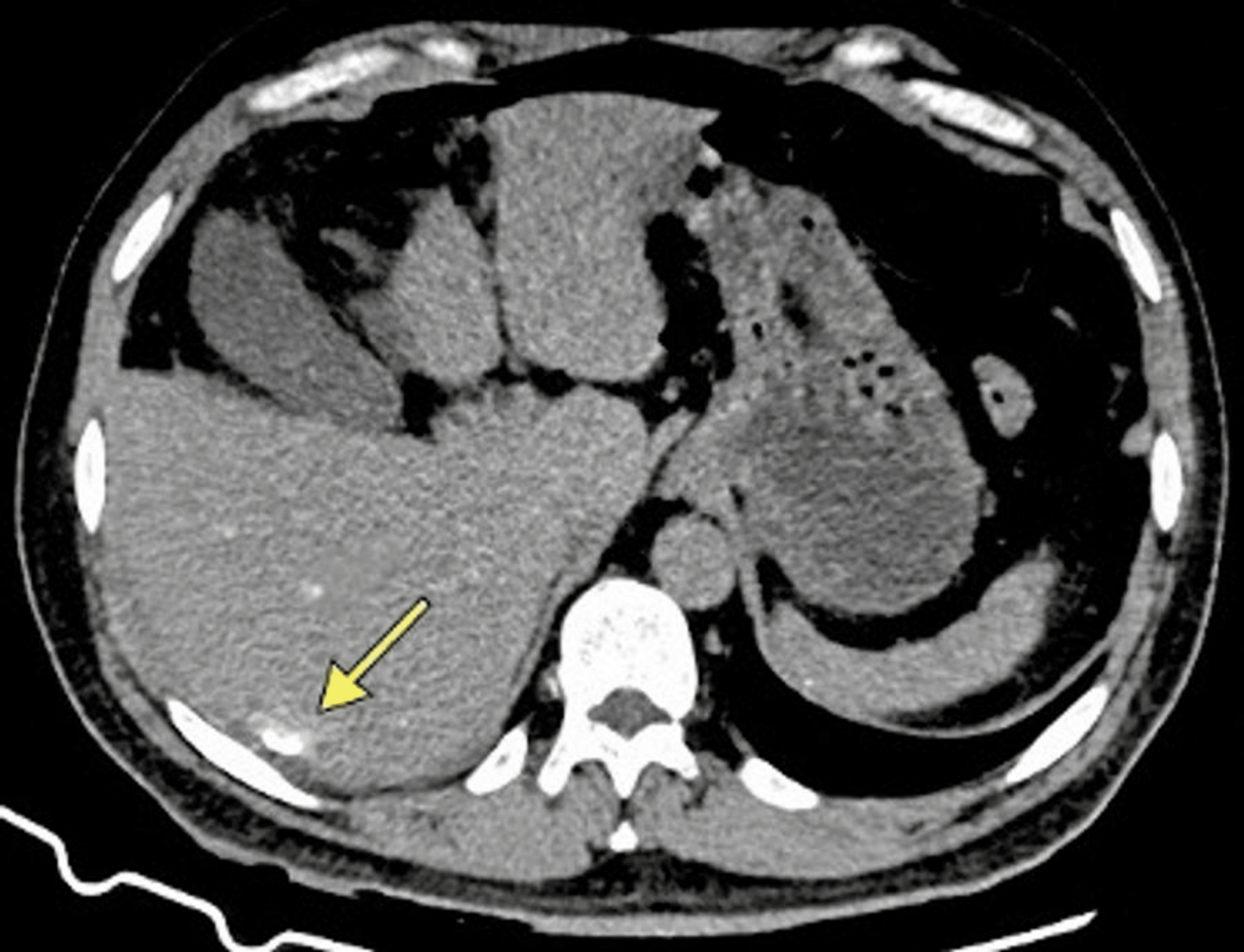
Preoperative contrast-enhanced abdominal CT Axial CT image obtained at emergency admission. The image demonstrates free intraperitoneal air compatible with a perforated hollow viscus. An incidental calcified cystic lesion is visible in hepatic segment VII (arrow), measuring 5.3 cm in maximal diameter, with peripheral rim calcification. Because the WHO-IWGE cyst classification is ultrasound based, any stage inference from CT alone should be interpreted cautiously, and formal ultrasound staging was not performed in this case. CT, computed tomography; WHO-IWGE, World Health Organization Informal Working Group on Echinococcosis.
